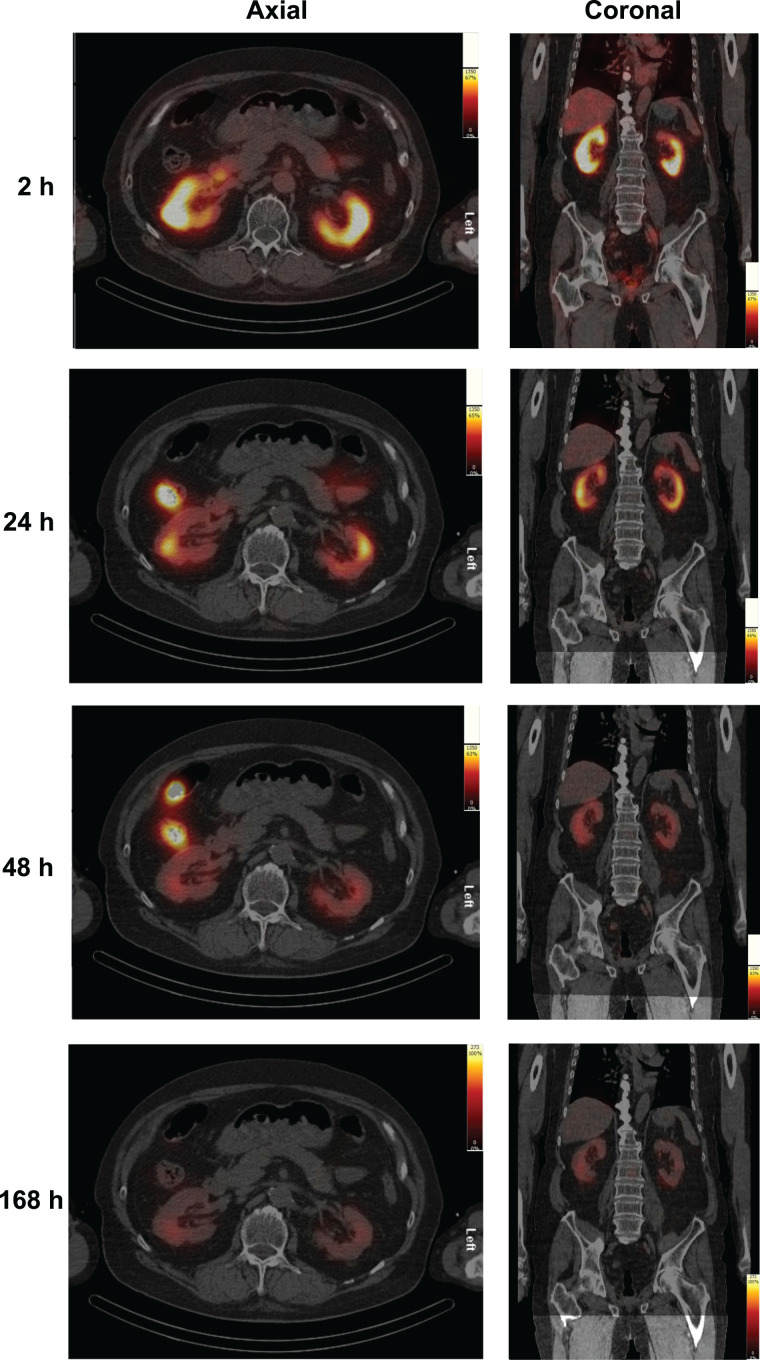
Representative SPECT/CT images show kidneys of a patient at various times during cycle 1 of ^177^Lu-PSMA-617 treatment. SPECT images (black/red/yellow scale) show uptake of ^177^Lu-PSMA-617 in kidneys (axial and coronal orientations) at 2, 24, 48, and 168 h during cycle 1. Underlaid CT images (gray) are scaled equally. Both image types are maximum-intensity projections.
